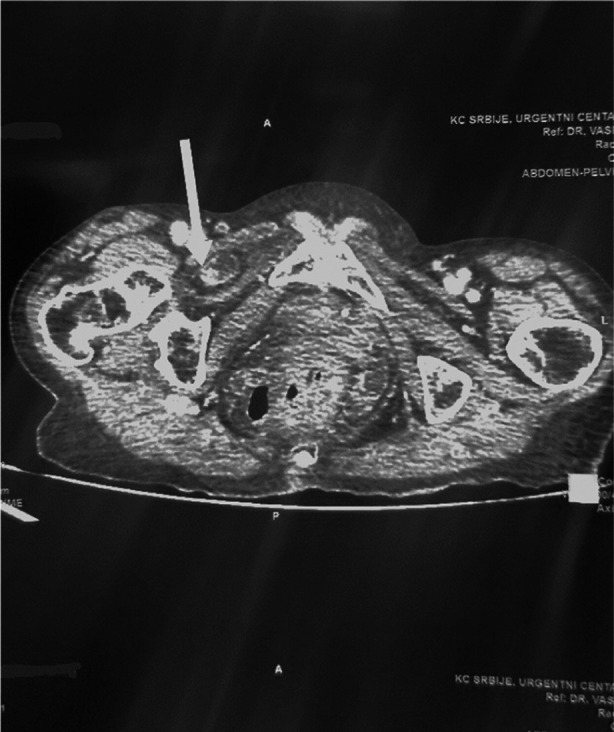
Incarcerated small bowel on CT (arrow).
